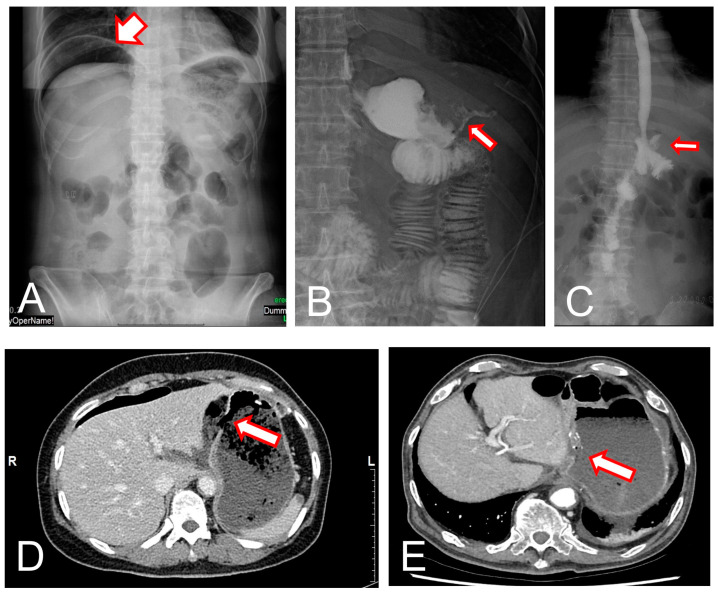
(A), Subphrenic pneumoperitoneum (arrow) that developed suddenly during the recovery period after surgery in simple abdomen X-ray. (B) Postoperative leak (arrow) showed in gastrojejunostomy site by upper gastrointestinal series. (C) Leak (arrow) showed in esophagojejunostomy site. (D,E) Defect (arrow) in the remnant stomach and food material around the stomach shown on an abdominal CT scan.
